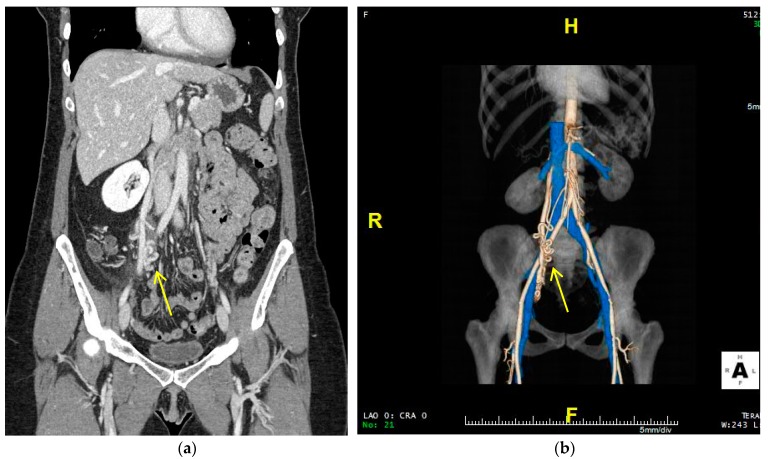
CT and computed tomography angiography (CTA) images of pelvic AVM. (a) The CT image on the left shows a conglomerate of tortuous vessels. (b) The CTA image on the right allows for better spatial resolution to visualize AVM morphology. Both modalities show a potential Cho–Do type II nidus with abnormal feeder connections from the infrarenal abdominal aorta and anterior division of the interior iliac artery and abnormal drainage to the right gonadal vein.
